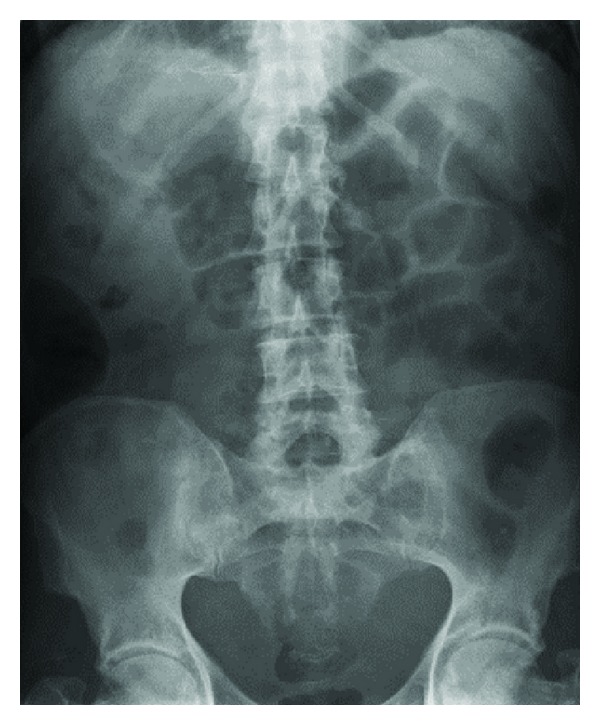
Plain abdominal radiography.
